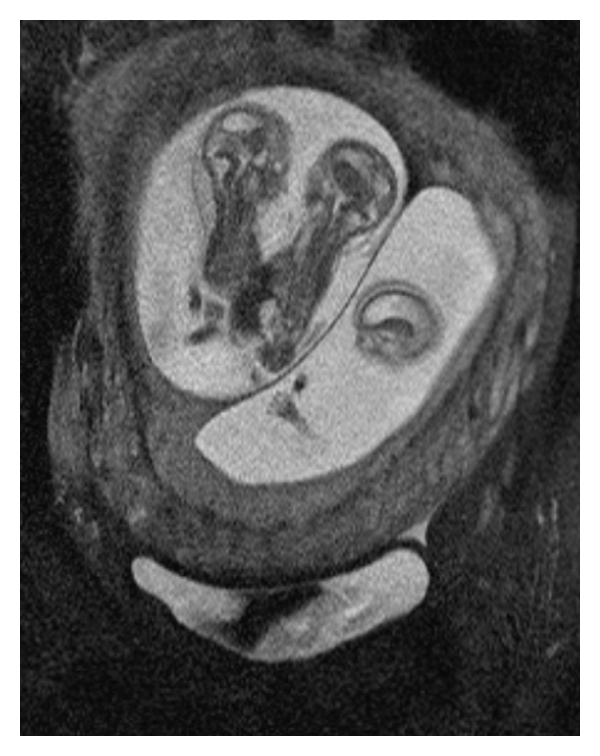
MRI images of conjoined twins in a triplet pregnancy.
